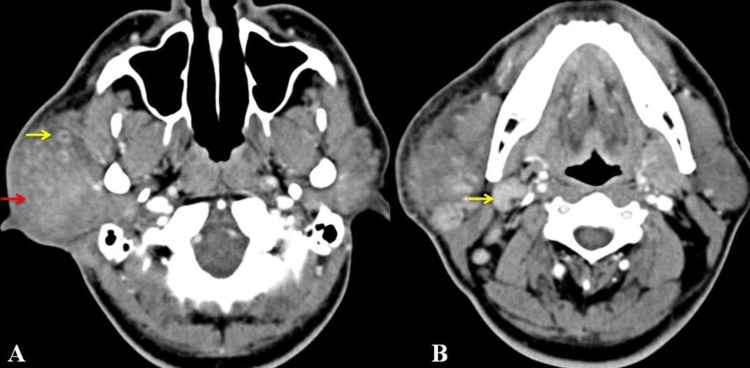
CT showing (A) enlarged left parotid gland (red arrow) with enhancing intraparotid lymph nodes yellow arrow and (B) enlarged neck lymph nodes (yellow arrow).
